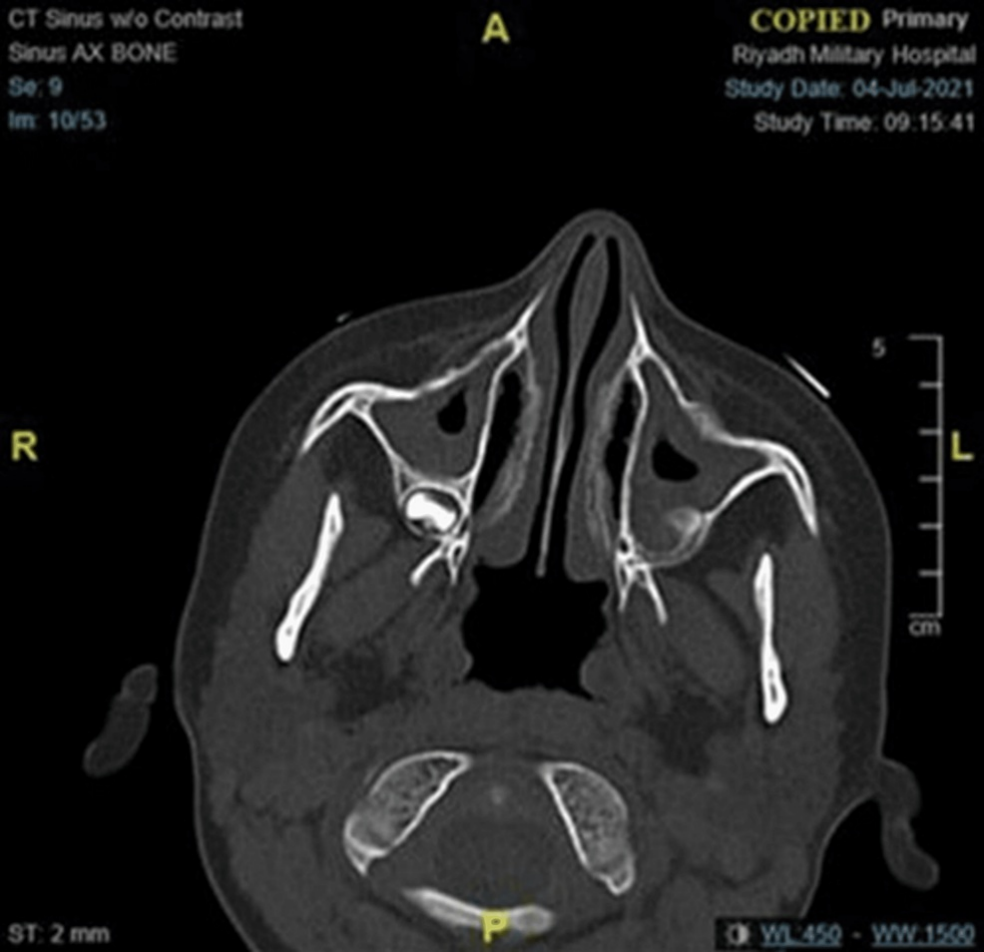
Sinus CT consistent with mucosal thickening of small sphenoid sinuses, ethmoid and maxillary sinuses; nasal septum is intact and middle ear cavities are well aerated
